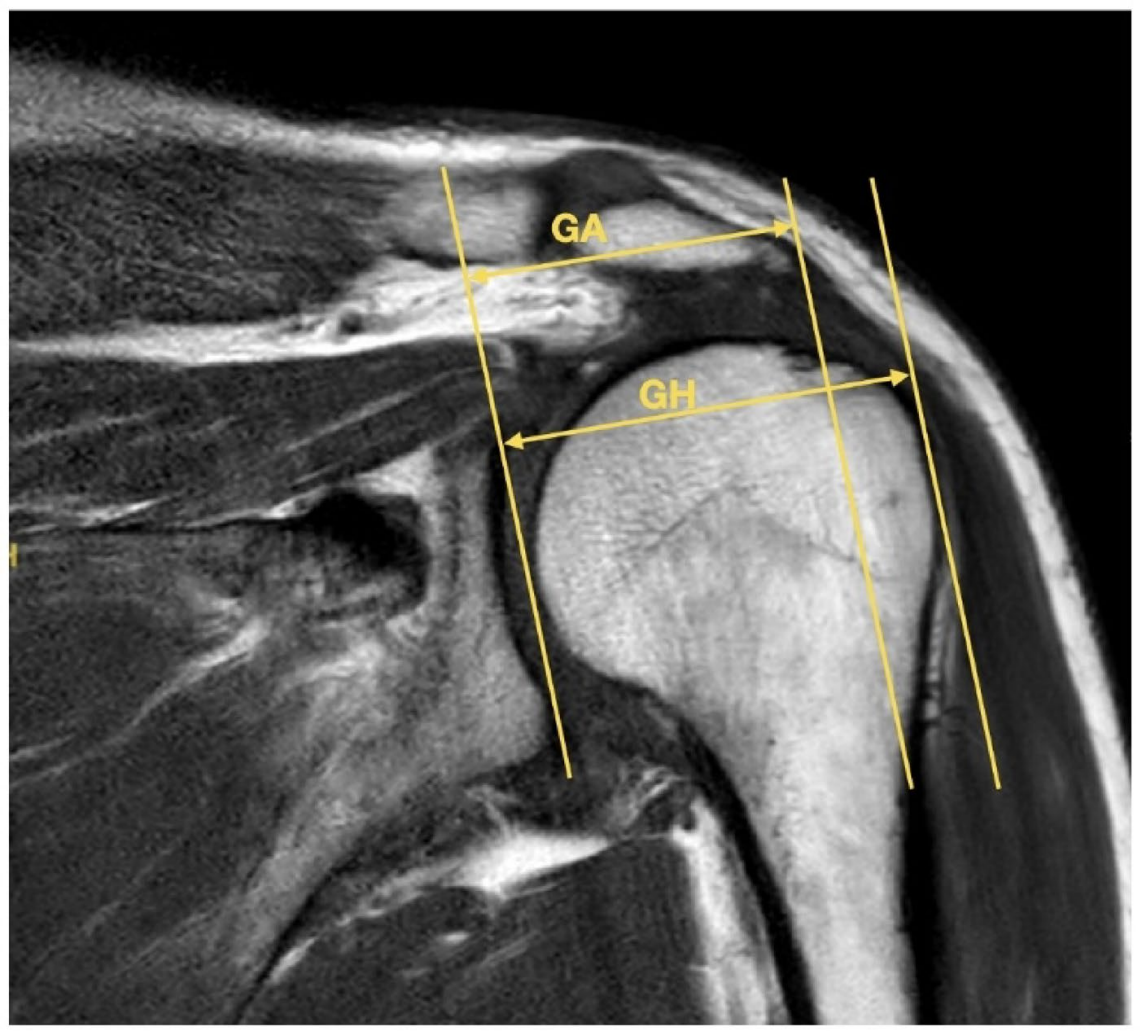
MRI assisted determination of the acromial index. This figure displays the method used to calculate the standard acromial index (AI) from MRI scans. Measurements were taken in the coronal plane, using the distance from the glenoid cavity to the lateral acromion and the distance to the lateral humeral head margin. The ratio of these two distances defines the AI. MRI offers high-resolution anatomical visualization, enabling precise identification of these landmarks in a static imaging context.
